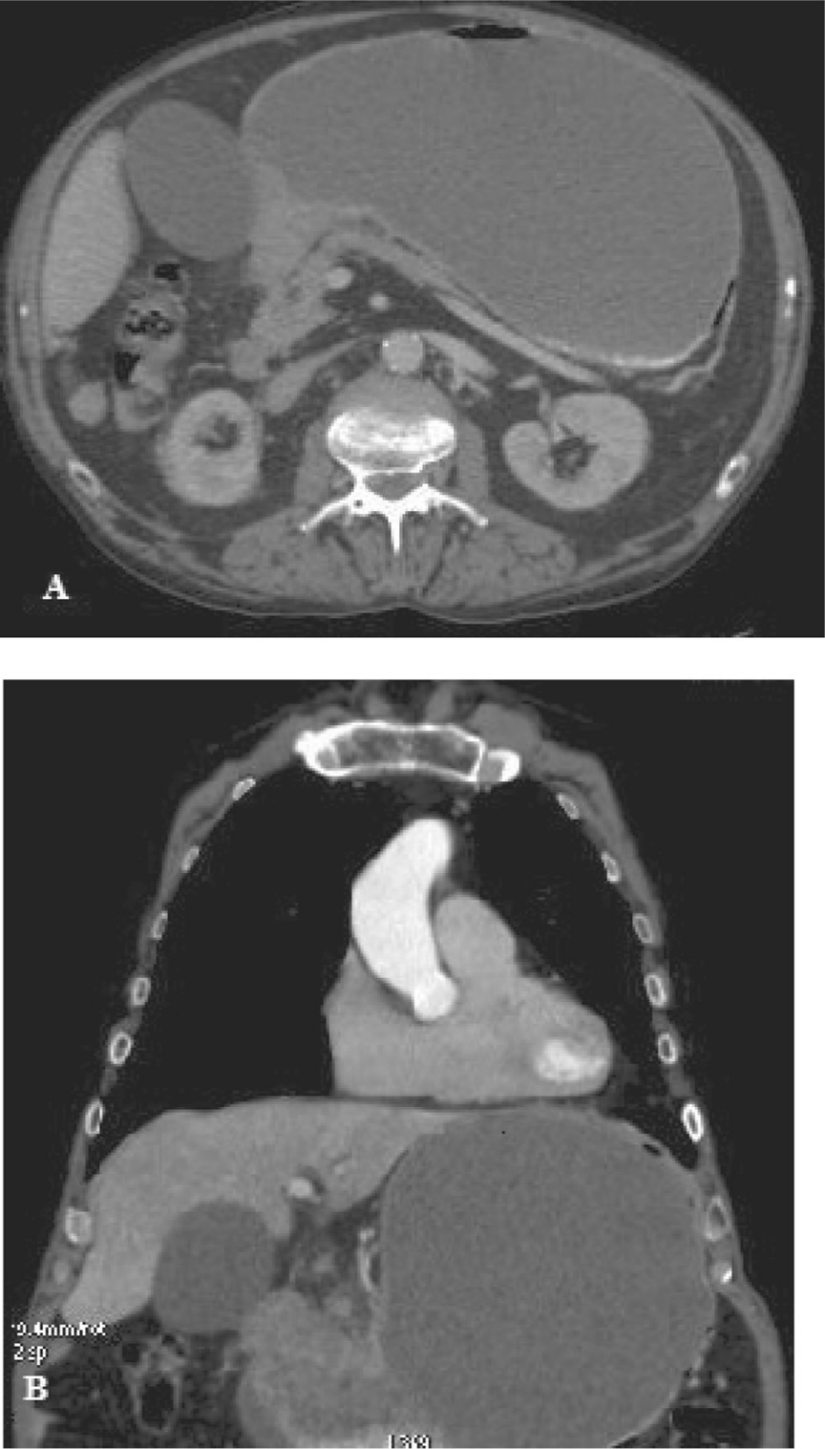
A 71-year-old man with antrum gastric cancer. A) Transverse axial contrast-enhanced CT image shows a wall thickening of the gastric antrum. B) Coronal reconstruction CT image shows irregularly wall thickening of the gastric antrum.
